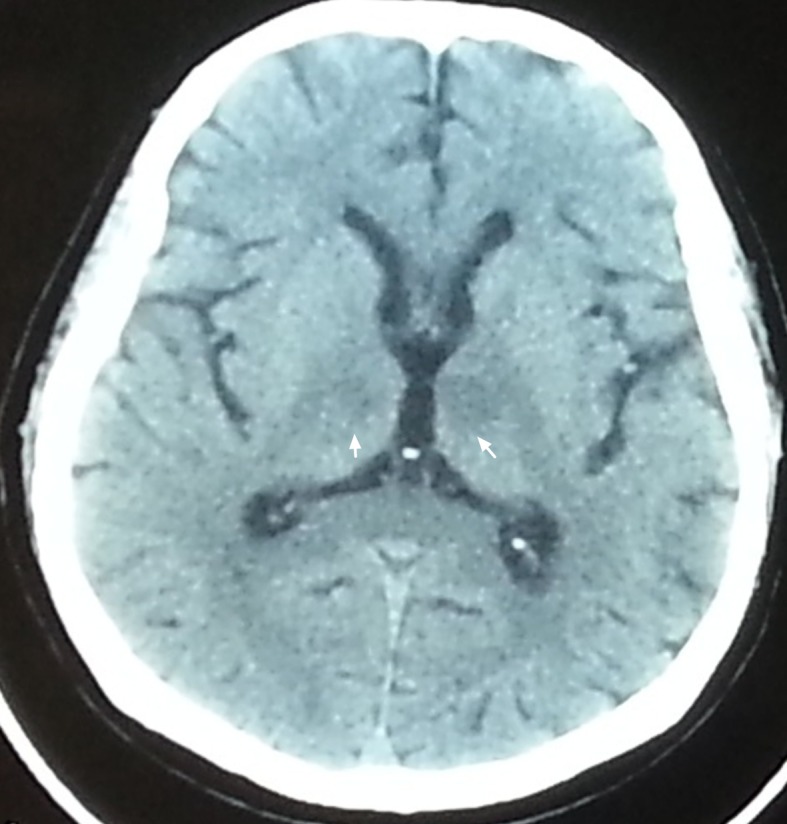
Axial non-contrast brain CT revealed faint hypodense lesion in bilateral paramedian thalamus (arrow).
